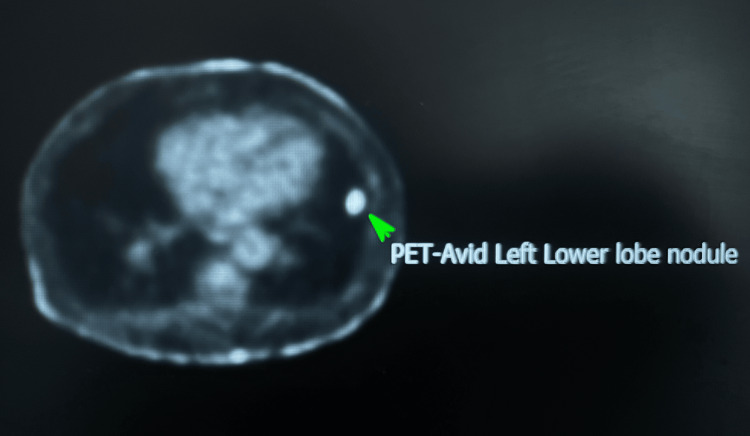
Intensely FDG-avid left lower lobe lung nodule measuring 15 mm (SUVmax 5.8), consistent with bronchogenic malignancy (stage T1b N0 M0). FDG, Fluorodeoxyglucose
